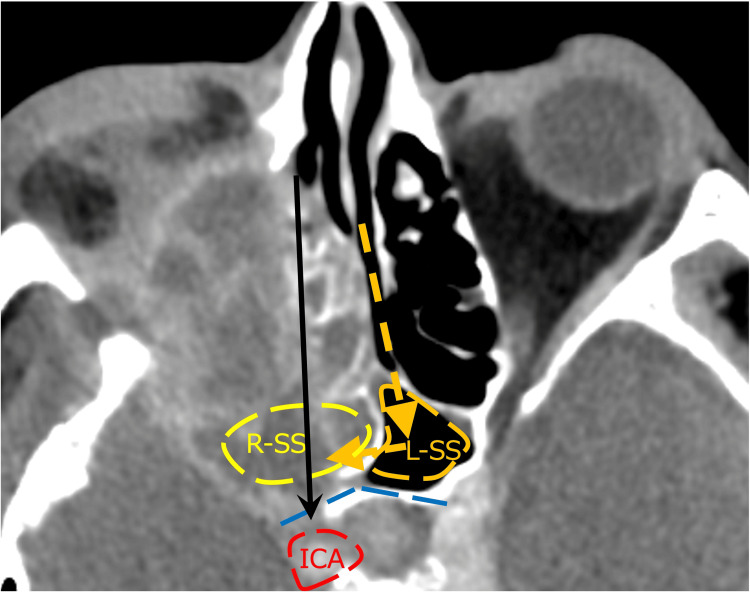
Contrast-enhanced CT of the sinus image (axial section) The right sphenoid sinus was obscured due to bone destruction. Additionally, the posterior wall of the right sphenoid sinus was destroyed, exposing the internal carotid artery. Therefore, performing debridement from the ethmoid sinus side posed a risk of internal carotid artery injury (arrow). By combining the transseptal approach with posterior nasal septum resection (dashed arrow), debridement of the ethmoid sinus side could be performed while keeping the posterior wall of the opposite sphenoid sinus (dashed line) under direct visualization, which allowed us to avoid injury to the internal carotid artery. R-SS: right sphenoid sinus; L-SS: left sphenoid sinus; ICA: internal carotid artery
